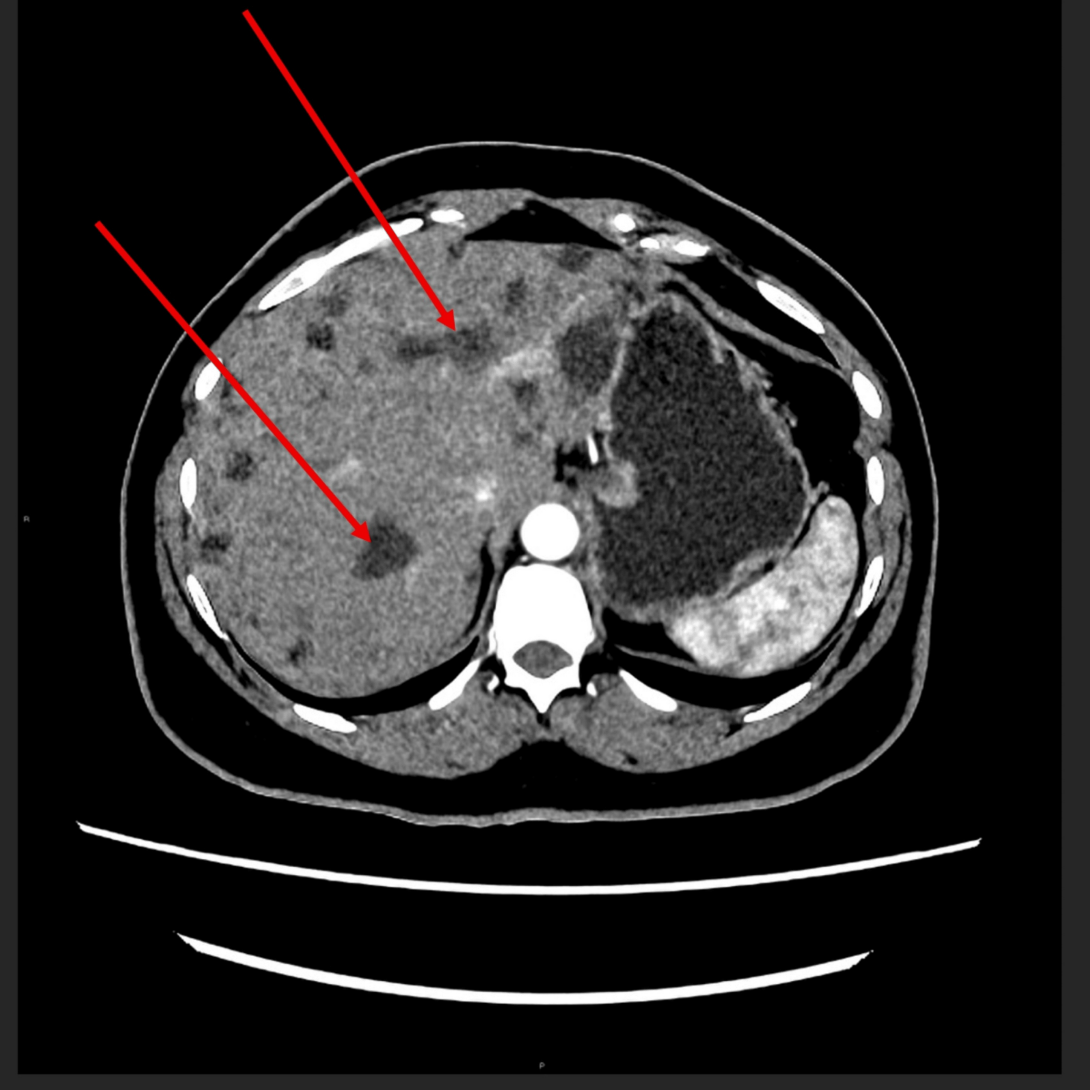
Multiple hypodense hepatic lesions (arrows) were revealed on the CT scan of the liver (arterial phase) CT: computerized tomography
